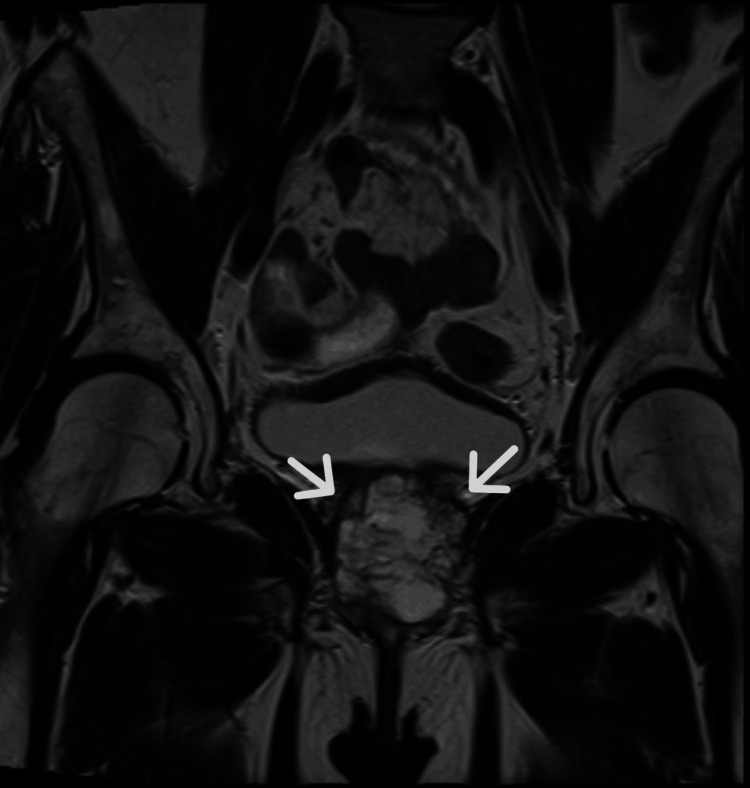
Coronal T2-weighted magnetic resonance image of a complex, multilobulated cystic periurethral mass denoted by the white arrows Often, this entity is described as "saddlebag" in appearance with relation to the urethra.
